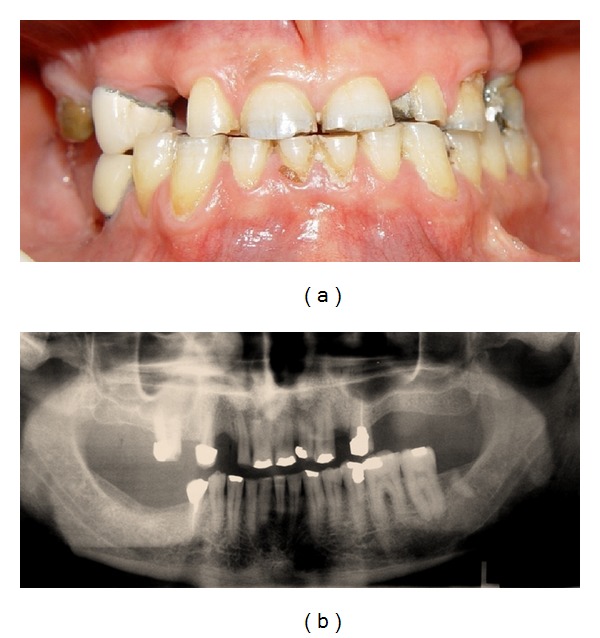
Frontal view of teeth in occlusion (a) and panoramic radiograph (b) before treatment.
